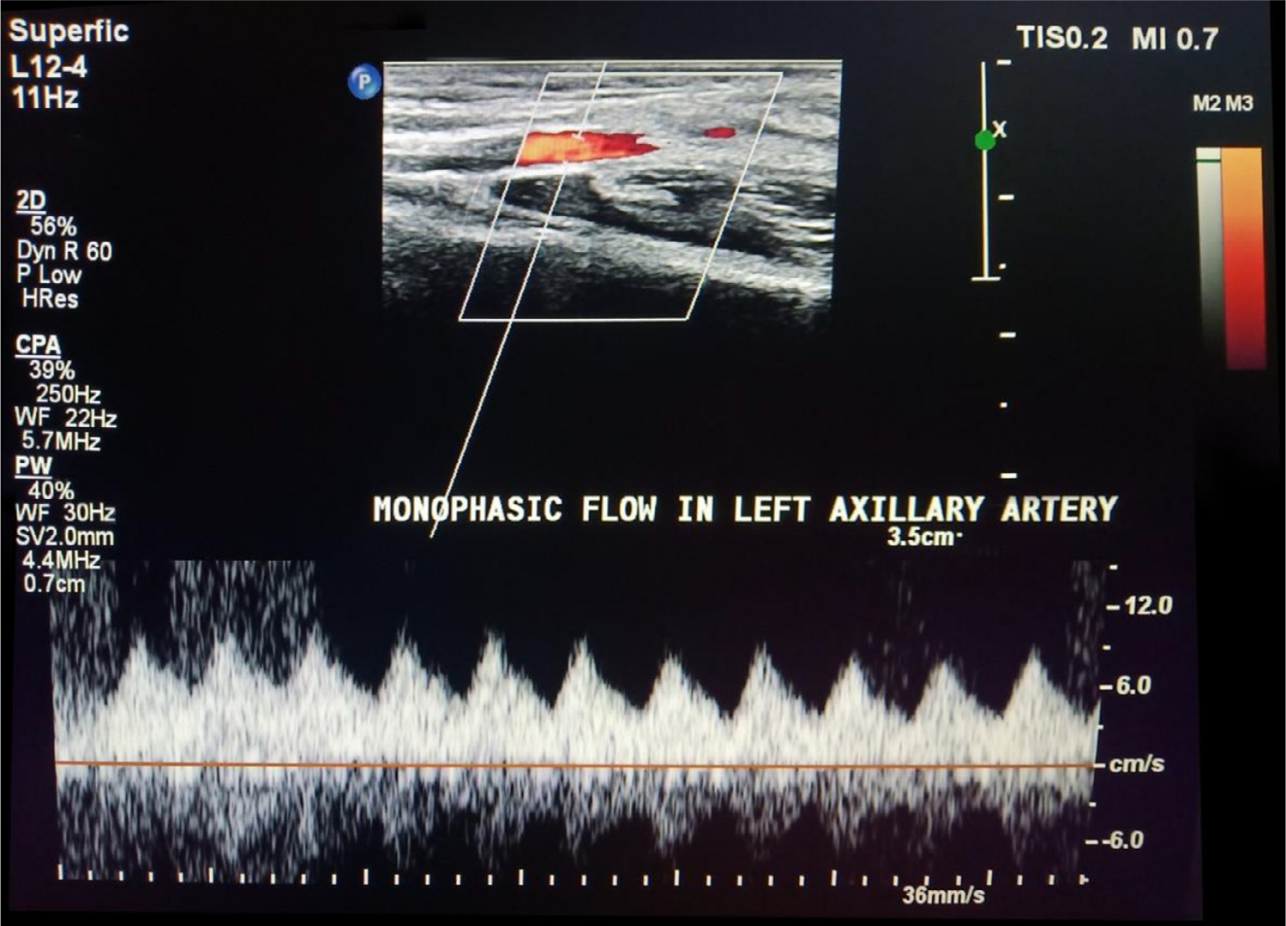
Spectral Doppler study of the left axillary artery.
